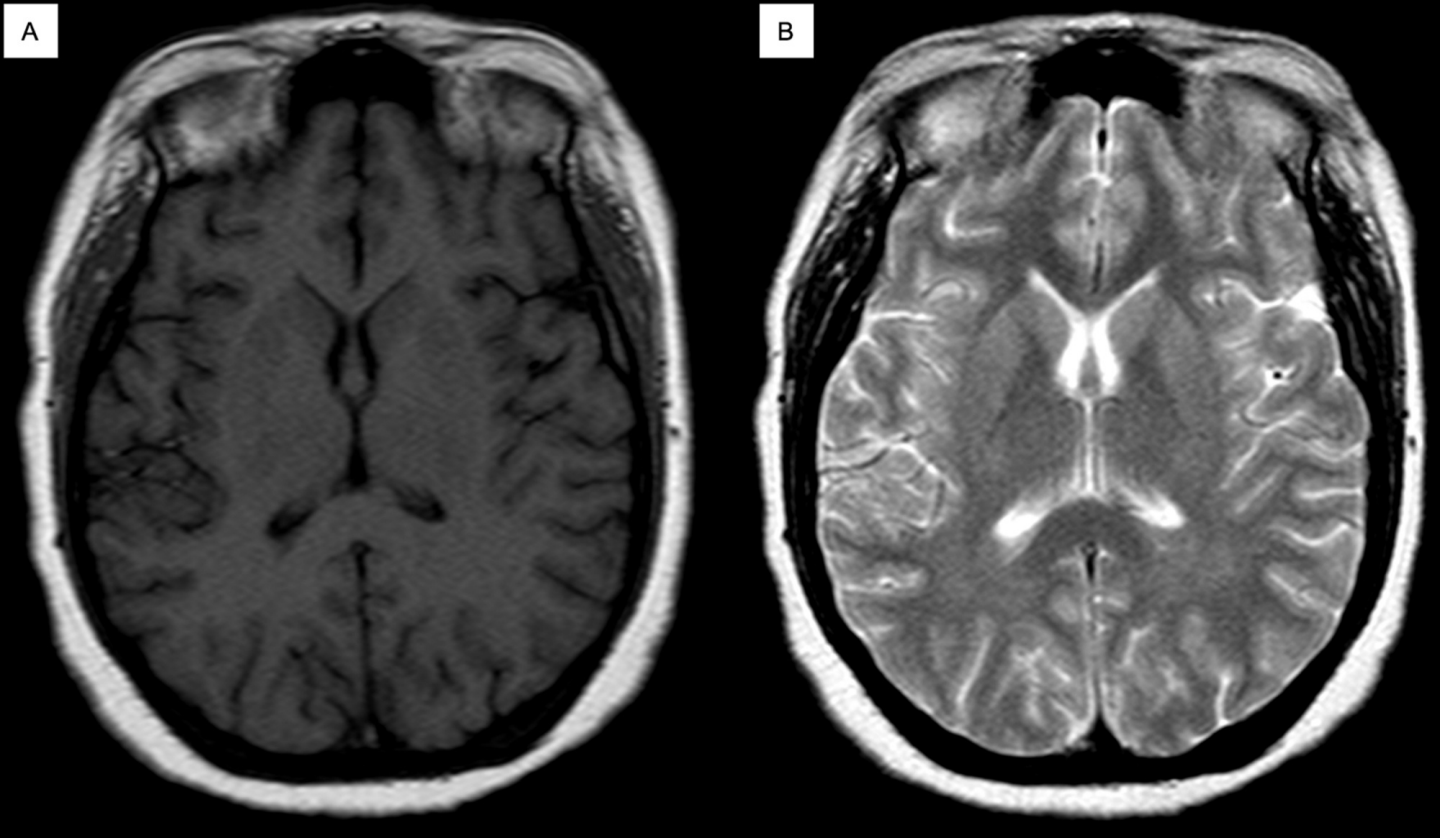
Case MRI images. (A) Axial noncontrast MRI in T1-weighted sequence without lesions in basal ganglia. (B) Axial noncontrast MRI in T2-weighted sequence without lesions in basal ganglia. MRI: magnetic resonance imaging.
